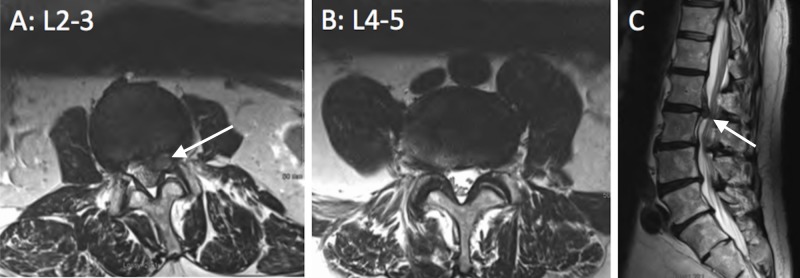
Lumbar Spine MRI 1A. L2-3 disc, axial T2 sequence through the L2-3 disc space showing a large left disc herniation with lateral recess and foraminal stenosis. 1B. L4-5 disc, axial T2 sequence through the L4-5 disc space showing minimal arthritic changes and no impingement of the exiting and passing roots. 1C. Sagittal T2 sequence slightly to the left of midline showing a large L2-3 disc herniation and only minor changes elsewhere. The intradural roots at the L4/5 level can be clearly visualized and are non-edematous and non-displaced.
